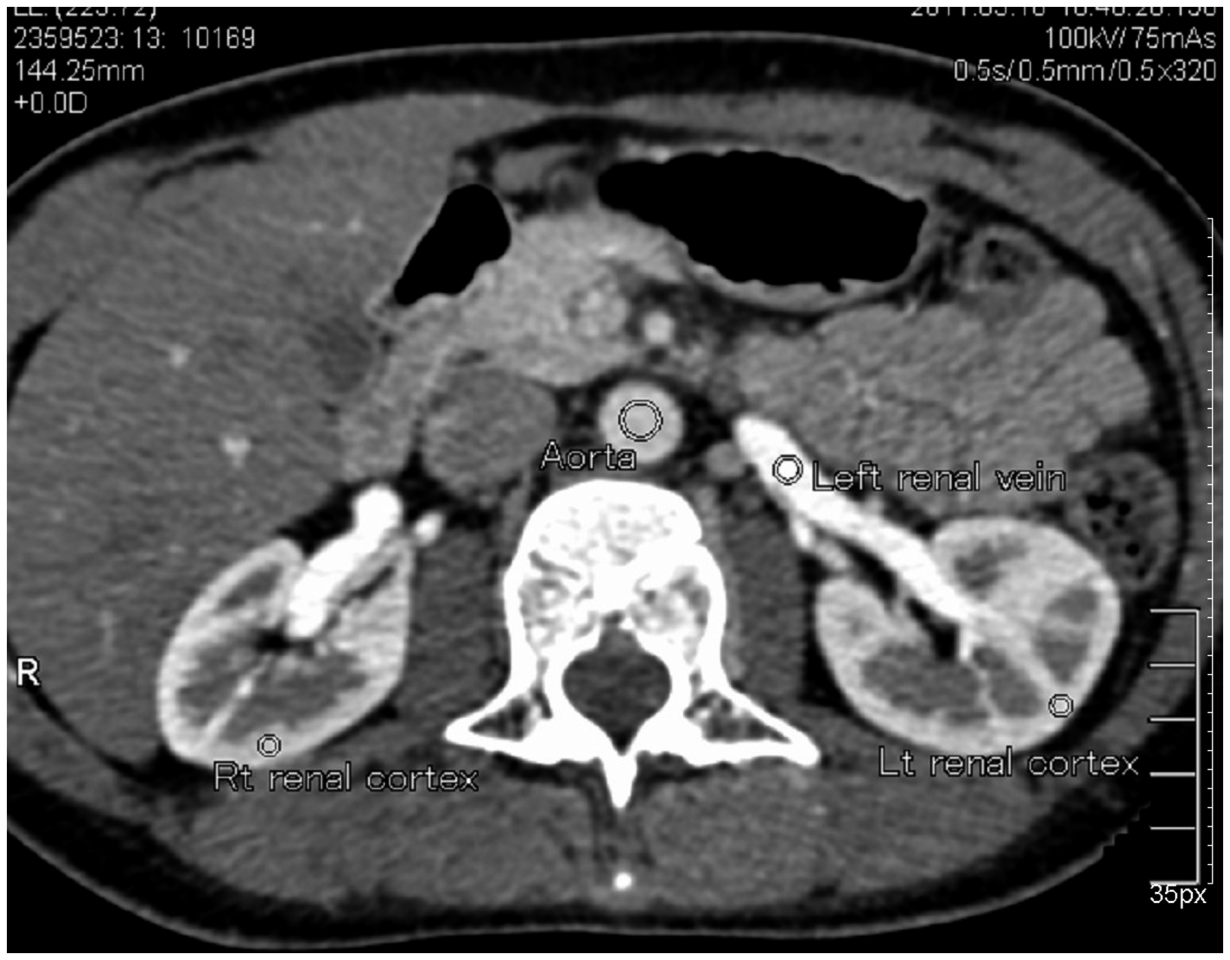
The CT perfusion regions of interest (ROI) included the aorta, left renal vein and bilateral renal cortices.
